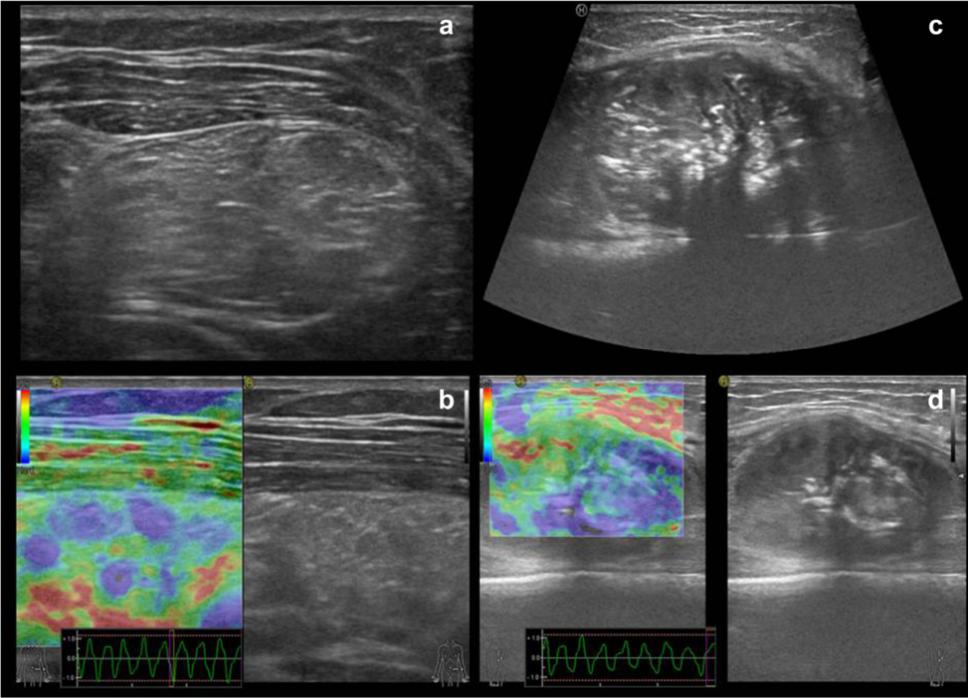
Ultrasound imaging. B-mode and elastosonographic evaluation of intramuscular lipoma (a, b) and osteosarcoma involvement of soft tissue (c, d), with calcifications included within the latter lesion. In the box of elastosonography (b and d) a colour map ranging from *blue* to *red* represents tissue elasticity; *blue* is associated with stiffness, *red* with softness
